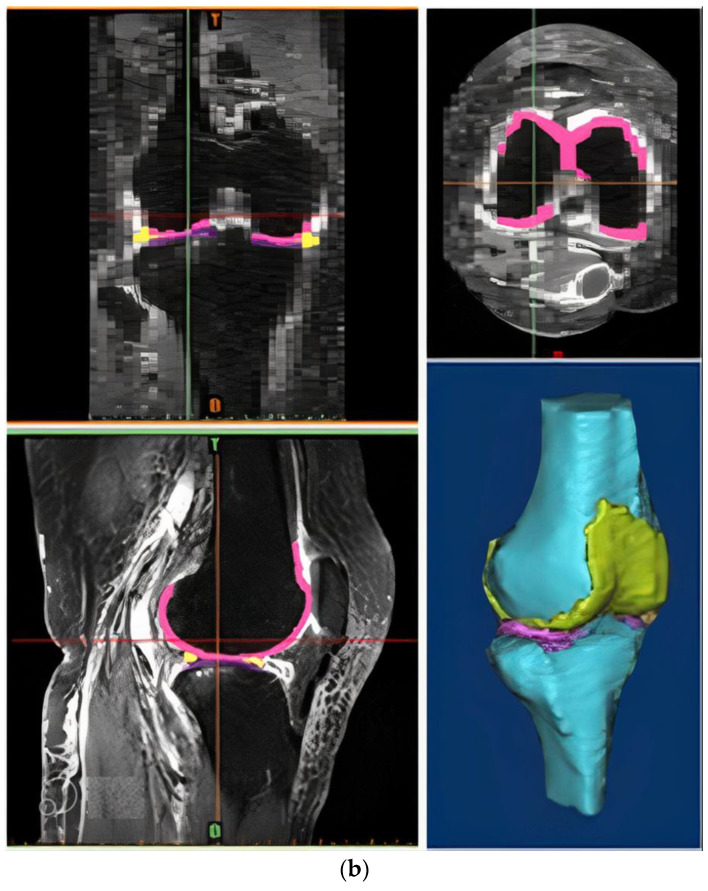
Data reconstruction in Mimics software (a) CT data (b) MRI data.
